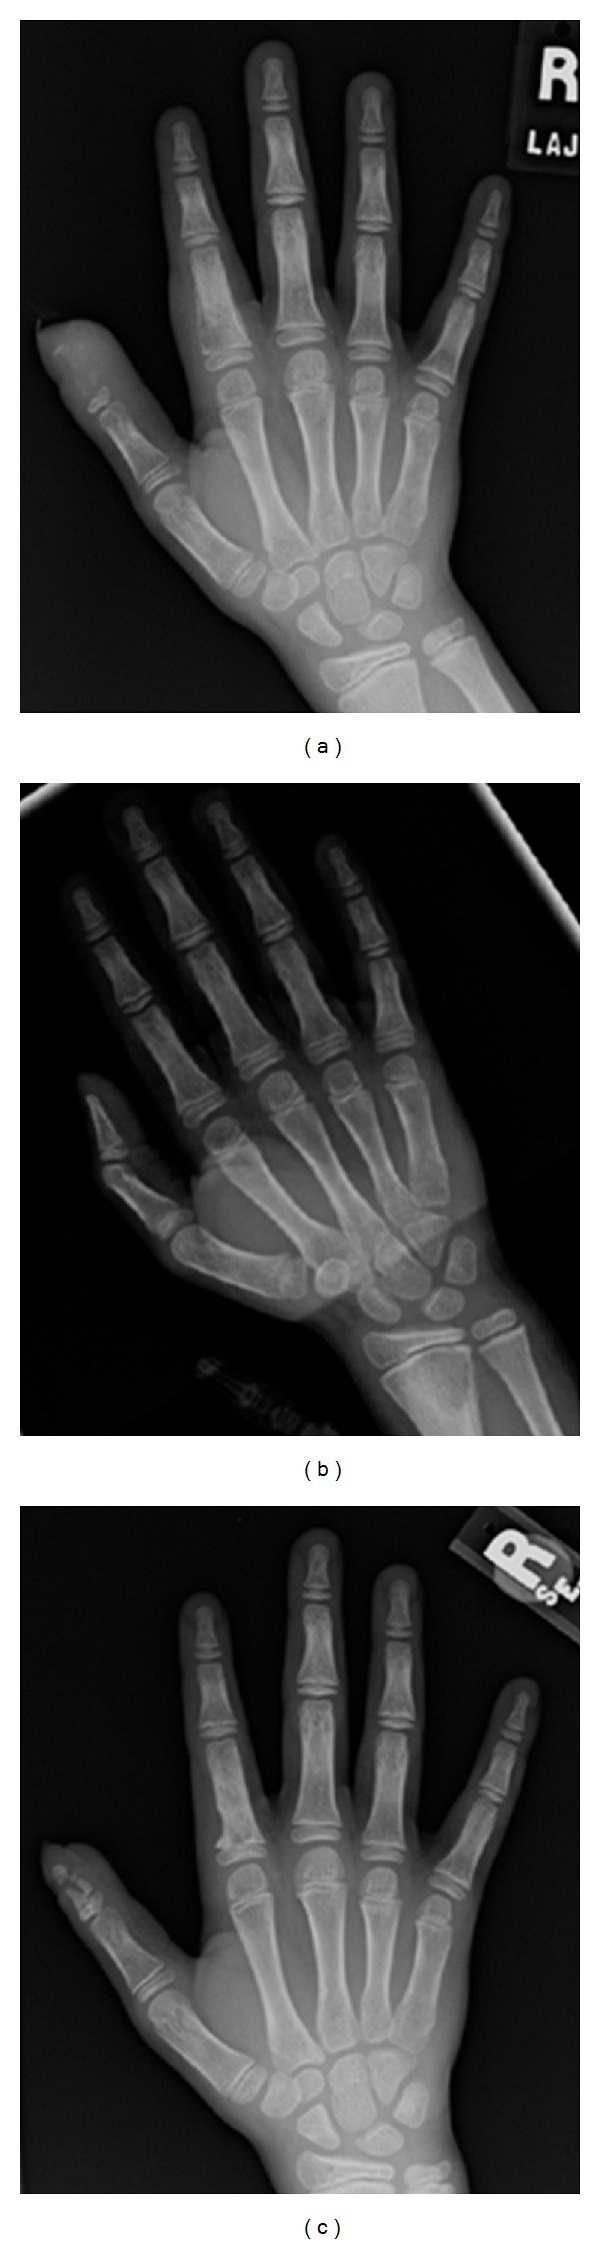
(a) Plain film of the right hand showing almost complete demineralization of the distal phalanx of the thumb and periosteal elevation and osteolysis of the proximal phalanx of the second digit. (b) Plain film obtained during skeletal survey after readmission, demonstrating an osteolytic lesion of the distal left radius. (c) Plain film of the right hand obtained after four months of antifungal therapy, demonstrating remineralization of the distal phalanx.
